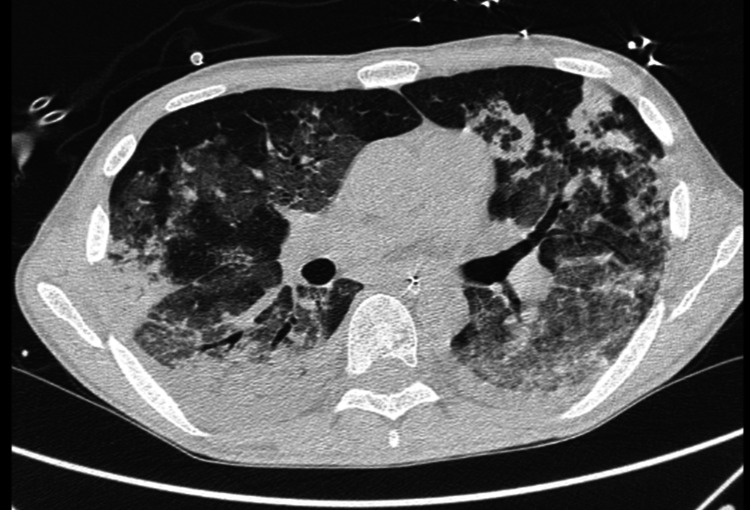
Pulmonary CT scan on day 22 after admission: SARS-COV-2 organizing pneumonia
